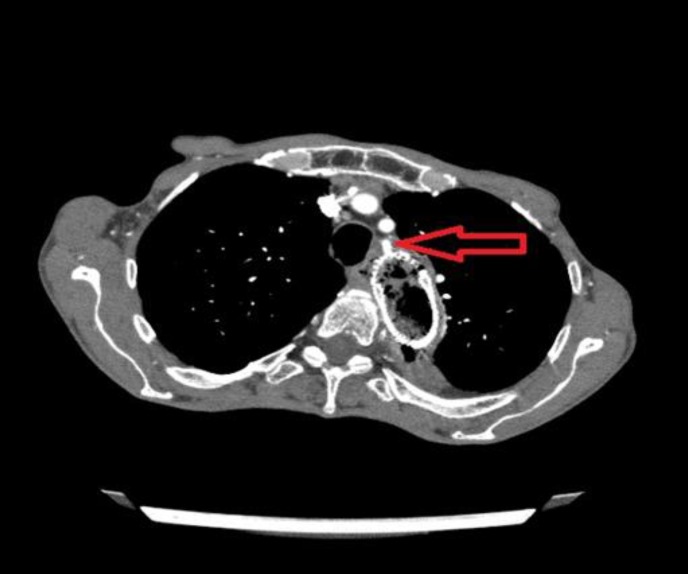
Computed tomography demonstrating an arterioesophageal fistula (red arrow) on the left subclavian artery where the esophageal stent touched
